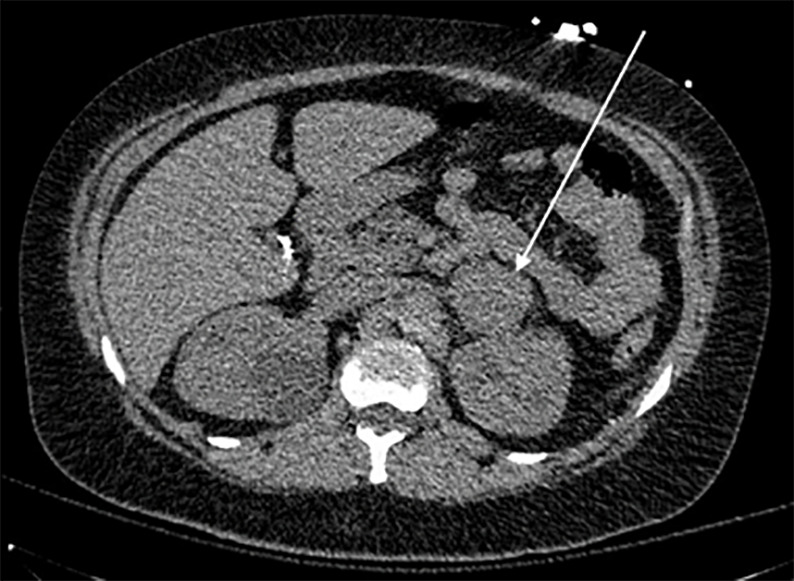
Computed tomography showing soft tissue density lesion measuring approximately 4 centimeters (cm) × 4.5 cm in the left suprarenal region.
